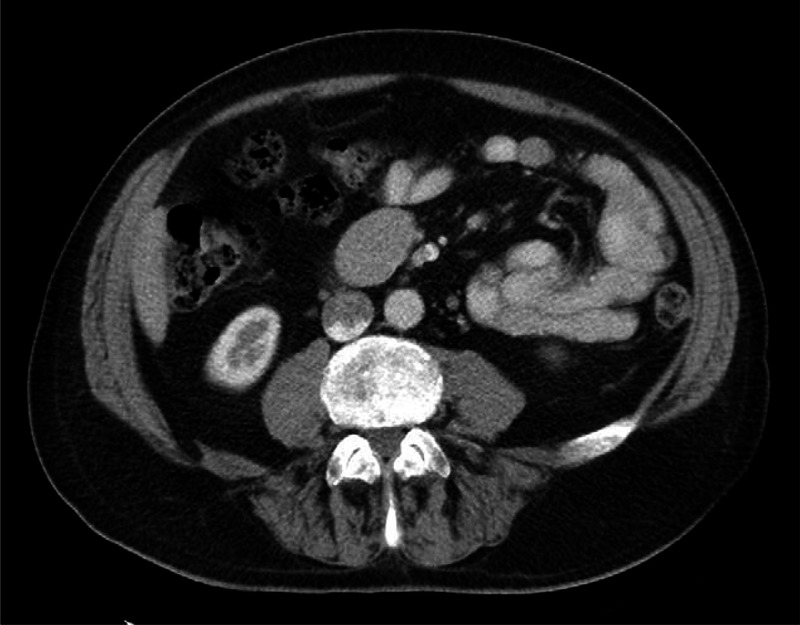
Abdominal computed tomography after administration of oral and intravenous iodinated contrast shows a homogenous enhancing mass in the root of the mesentry. No internal calcification or necrosis.
